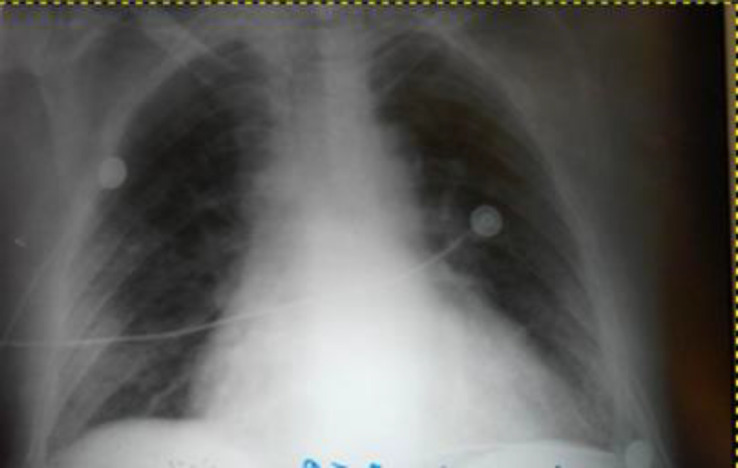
Chest radiography showed cardiomegaly, occlusion of the left phrenic-costal sinus and bilateral interstitial abnormalities
